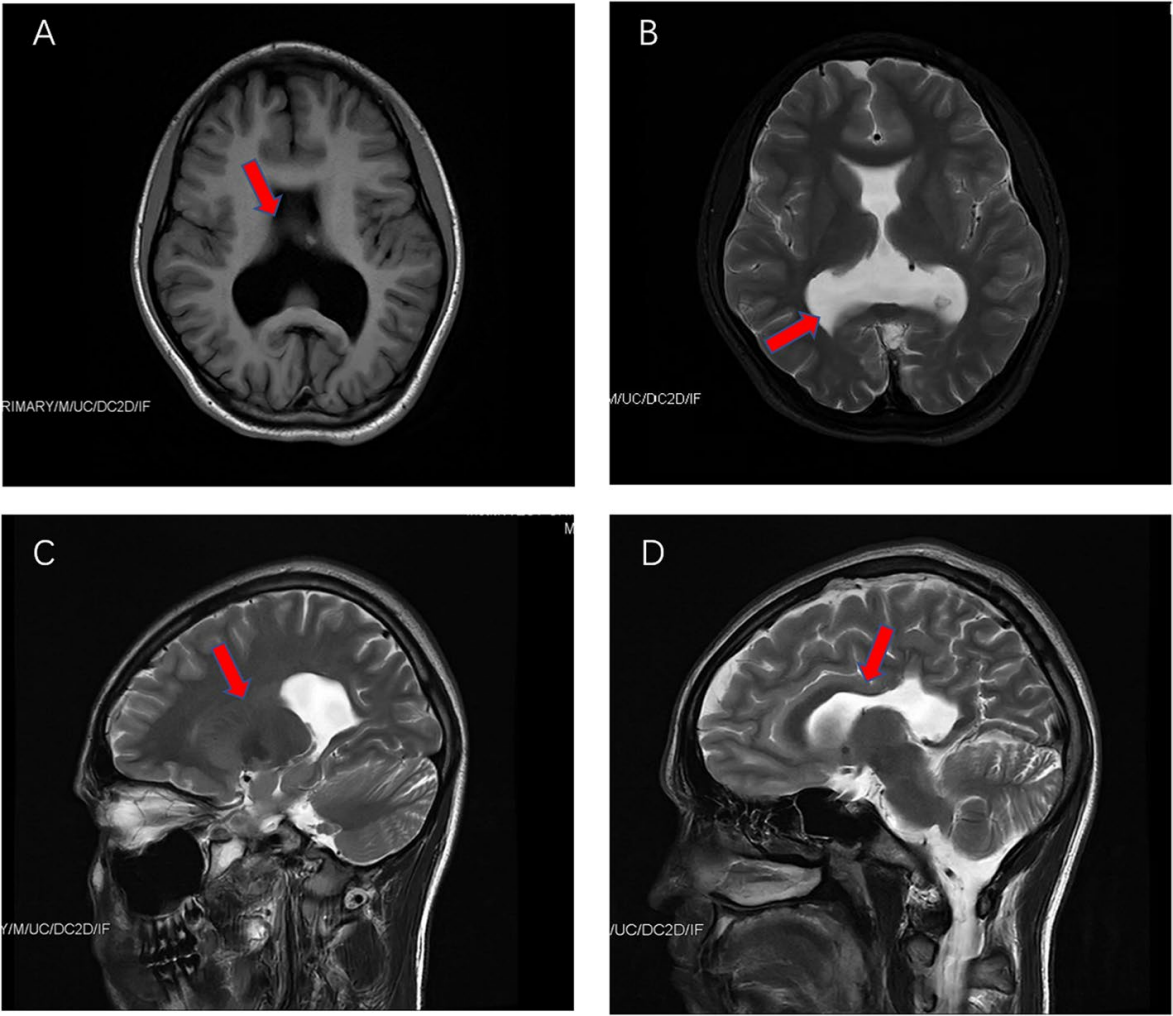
Brain MRI examination of a 17-year-old boy on admission. A and B axial T1-weighted images and T2-weighted images, showing absent septum pellucidum, connected lateral ventricles, and enlarged pedunculus posterior. C and D sagittal T2WI FLAIR images, presenting partial defect of the body of corpus callosum. And the cerebral convolutions adjacent to the absent corpus callosum region enlarged and shifted downward
